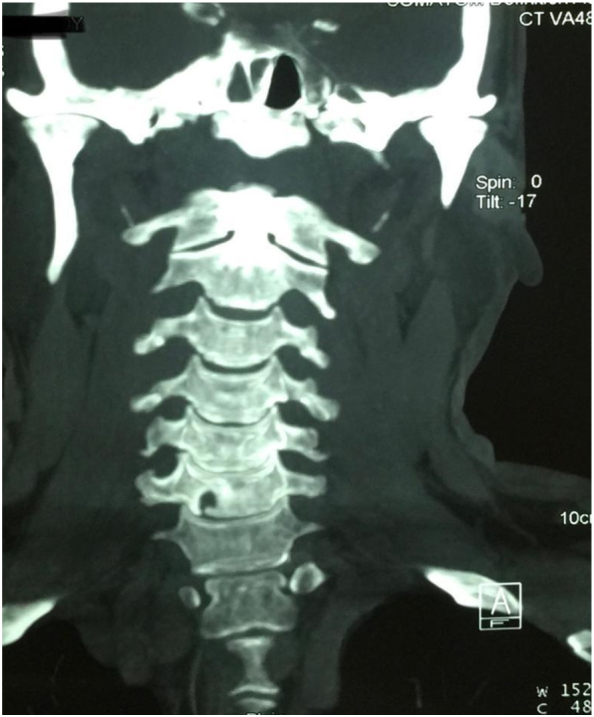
CT scan of cervical spine showing drill hole at C6 for C6/7 right lateral disc herniation.
